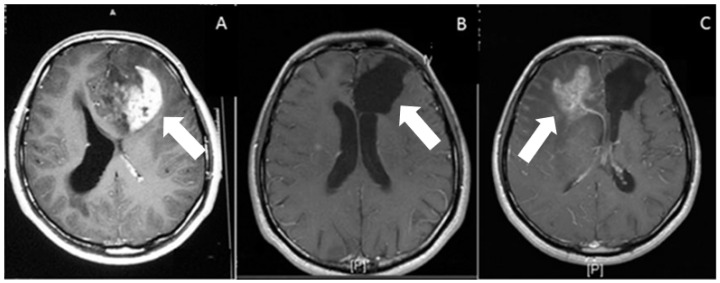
Post gadolinium contrast administration, T1-weighted axial images. (A) Preoperative, heterogeneous irregular enhancement, associated with the left frontal-lobe glioblastoma (arrow). (B) Postoperative (at 1 month) axial weighted image. On postoperative image, there is no residual enhancement. Arrow shows operation cavity. (C) Postoperative (at 18 months) axial weighted image shows recurrence of the tumor (white arrow) on contralateral hemisphere, associated with peripheral edema.
